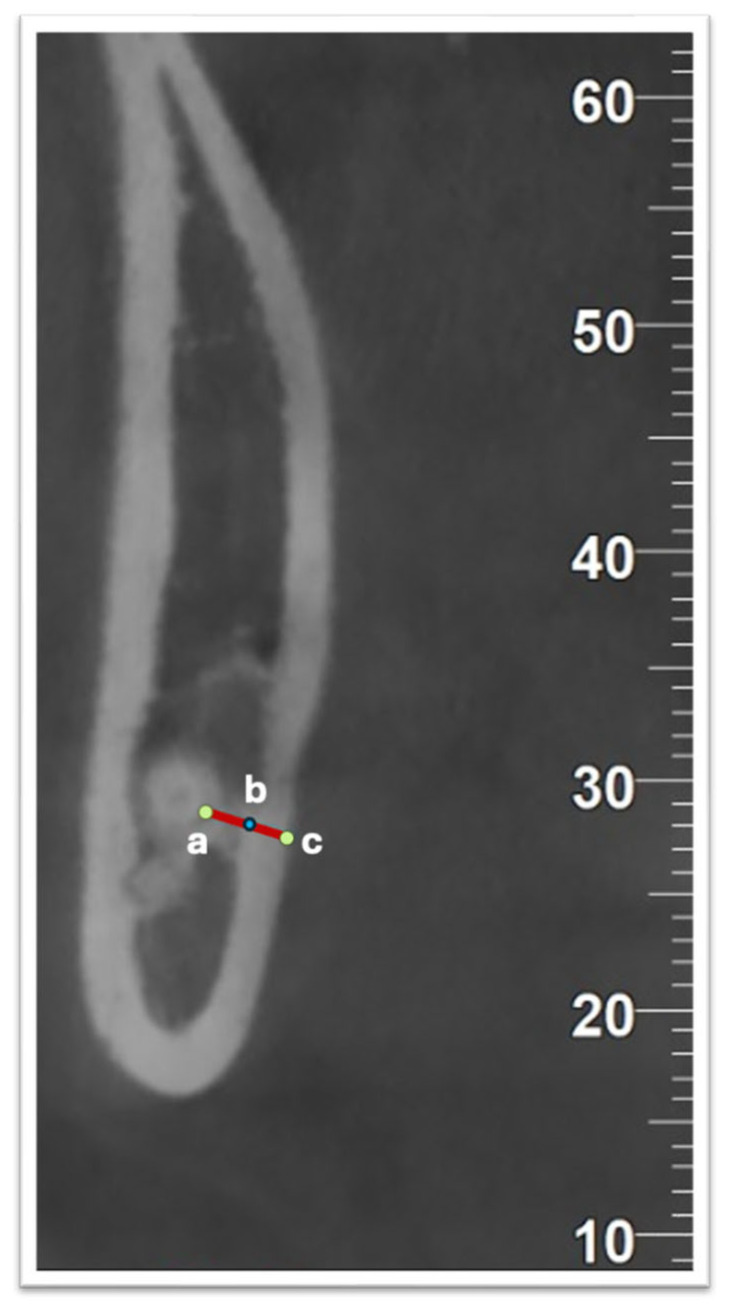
CBCT cross-sectional image demonstrating measurement methodology. Point a represents the apex of the root located most lingually, point b the inner surface of the lingual cortical plate, and point c the outer surface of the lingual cortical plate. The b–c line indicates the thickness of the cortical lingual bone, while a–c represents the distance from the root apex to the outer surface of the lingual plate.
